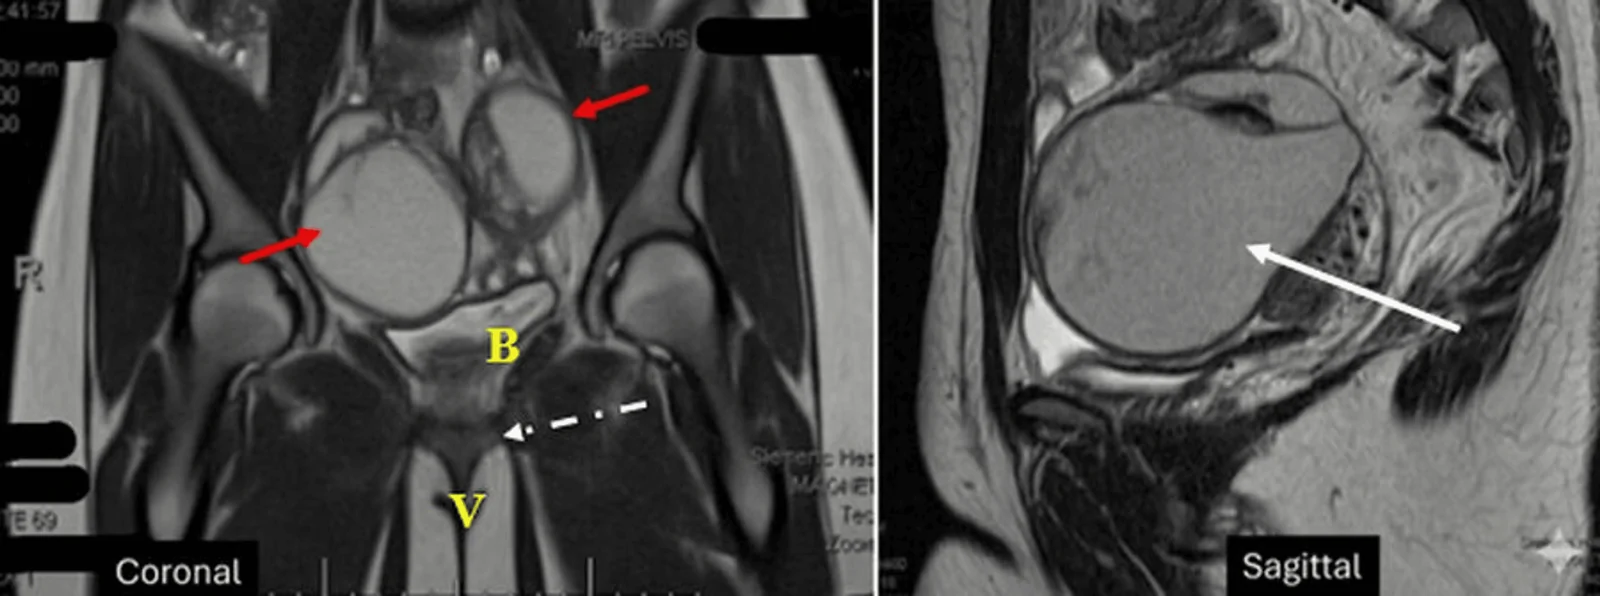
T2-weighted pelvic MRI demonstrating suspected outflow obstruction Coronal scan demonstrating possible bilateral hematosalpinx (red arrows) versus endometriomas. "B" indicates the bladder. The findings are suggestive of cervical hypoplasia versus agenesis; however, this image alone is insufficient to diagnose cervical agenesis. The absence of a clearly visualized cervical structure in this coronal slice may be attributable to severe anatomic distortion and the limitations of a single two-dimensional plane. Given the abrupt caliber change at the level of the possible internal os and the upper vagina (dotted white line), associated septa or webs cannot be completely excluded, although manual examination revealed no vaginal anatomic abnormality. The sagittal scan demonstrates possible hematometra (white arrow). MRI: magnetic resonance imaging
